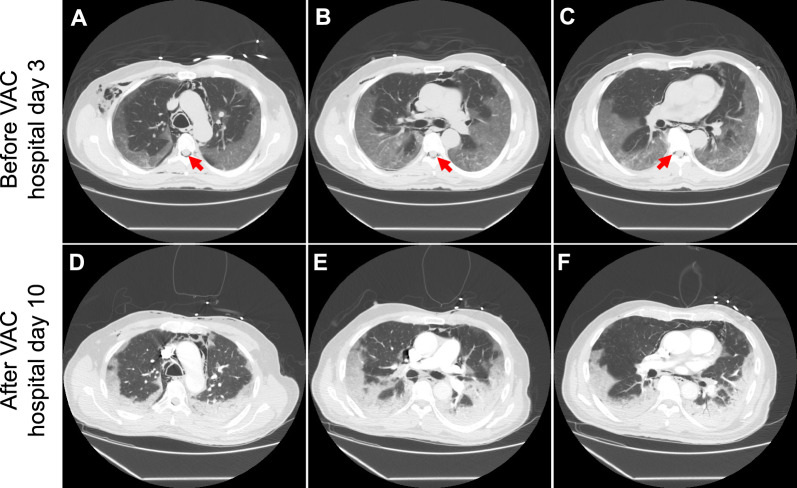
Computed tomography of the chest obtained before (A–C, on hospital day 3) and after vacuum-assisted closure therapy (D–F, on hospital day 10). (A–C) The images were obtained on the day when the patient developed chest pain and a “popping sensation” in the neck, showing extensive subcutaneous emphysema and pneumomediastinum. Gas tracks along the right pleural space. Epidural gas is also seen within the thoracic spine (red arrows). (D–F) The images were obtained on hospital day 10 to rule out pulmonary embolism when the patient developed worsening hypoxia, while on vacuum-assisted closure therapy. The overall decrease in the volume of soft tissue emphysema can be appreciated compared with (A–C). Significant bilateral consolidation compatible with coronavirus disease 2019 infection appears similar on the two sets of images
